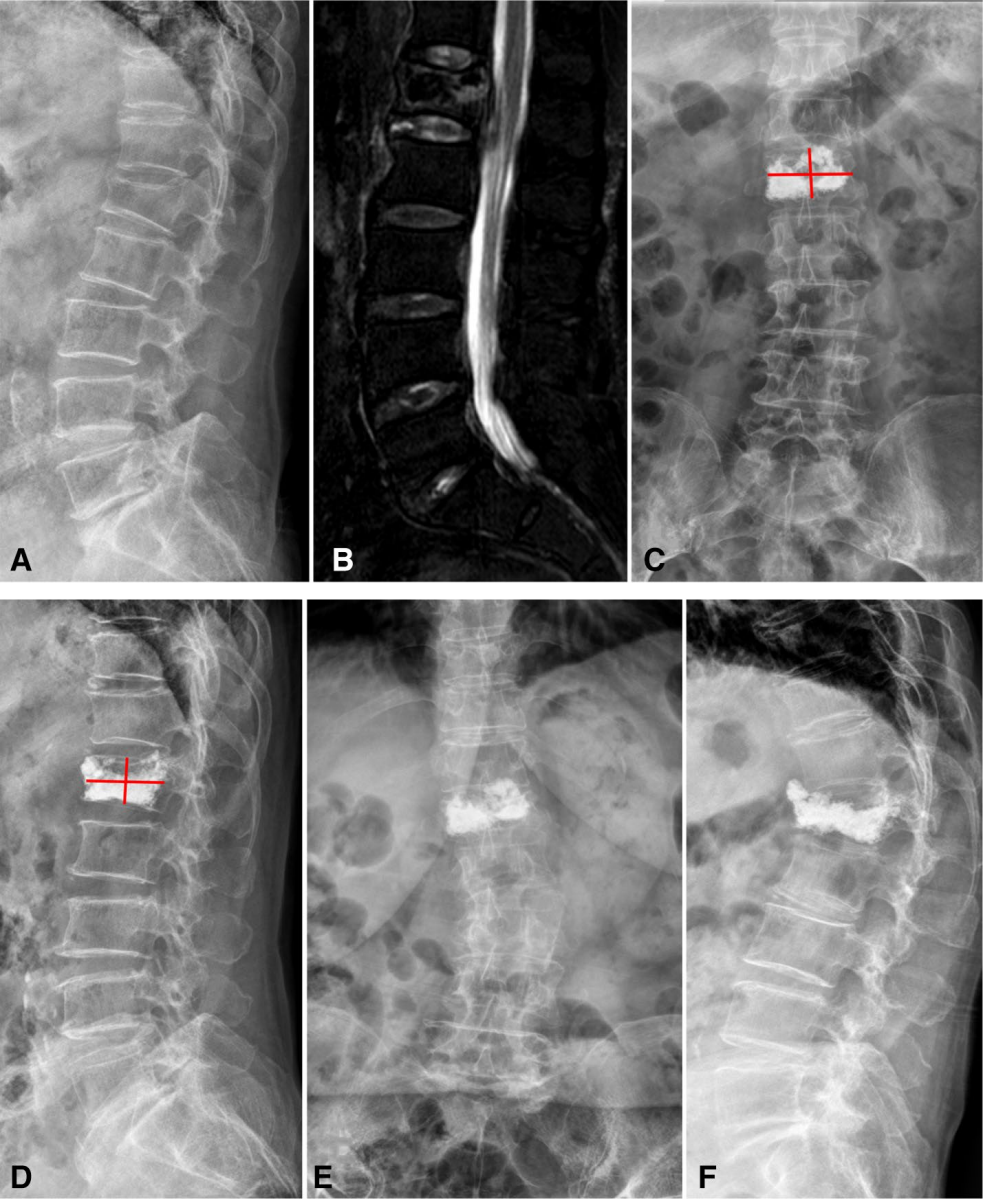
X-ray images of a 79-year-old woman. (A,B) Images taken at admission, showing vertebral compression fracture at L1. (C,D) Anteroposterior and lateral images taken after percutaneous kyphoplasty, showing restoration of the fracture. Bone cement distribution was scored as 7 using method 1, 8 using method 2, 6 using method 3, and 9 using methods 4 and 5. (E,F) Images taken at 6 months after surgery, showing re-collapse of the operated vertebra.
